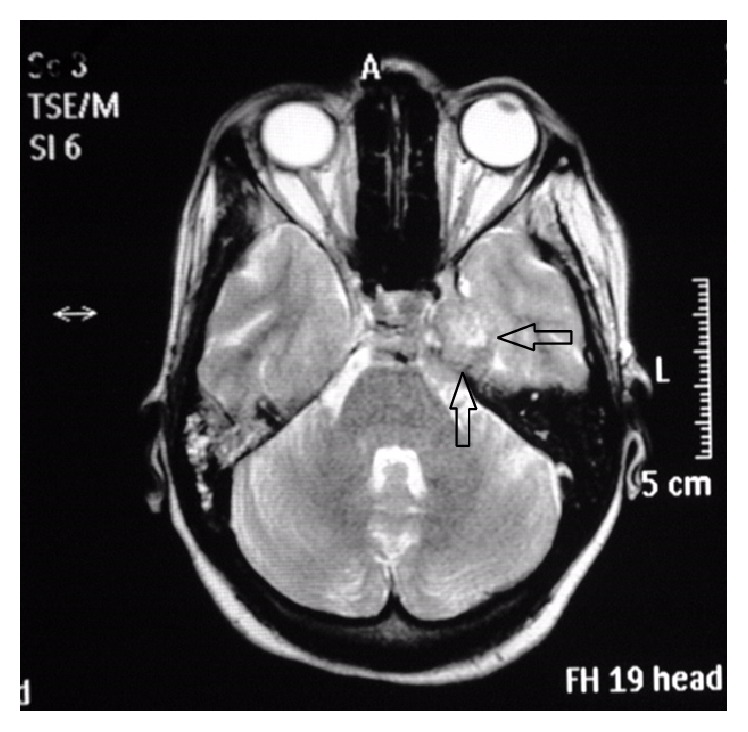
MRI postcontrast showing altered signal intensity of the clivus, axial section that appears heterogeneously hyperintense.
